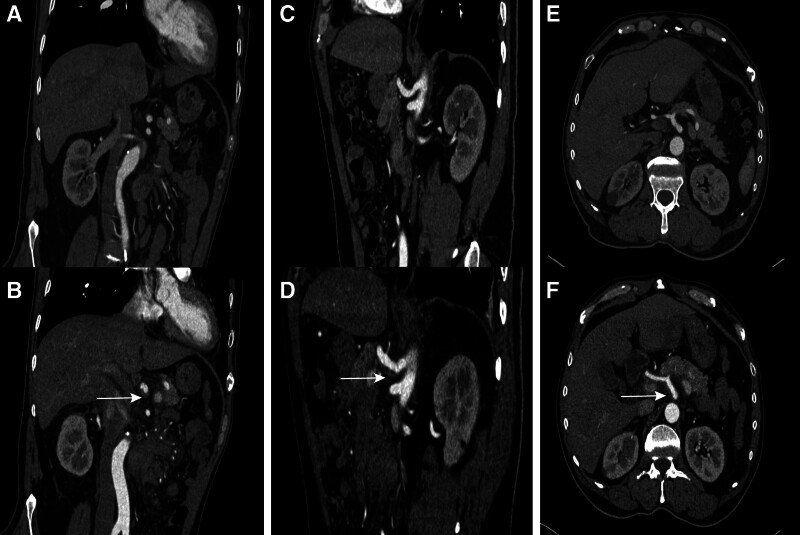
Contrast-enhanced CT scan. From left to right are the comparative images in the coronal, sagittal, and axial planes. Images (A, C, and E) represent the normal images before medication, while images (B, D, and F) represent the abnormal images after medication. Thin white arrows indicate the location of the celiac trunk AD. AD = aortic dissection.
